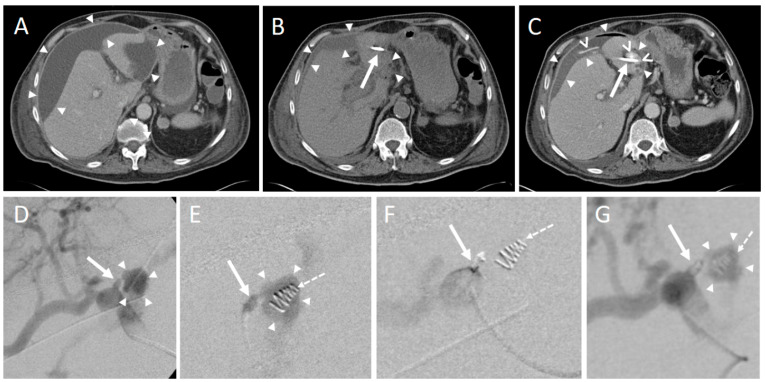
CT-guided drainage placement with a subsequent major complication according to SIR. (A) 71-year-old male underwent pancreaticoduodenectomy (PD) due to ductal pancreatic adenocarcinoma. Sixteen days later abdominal revision surgery with hematoma evacuation was performed due to arterial hemorrhage from the hepatic artery and small bowel segment resection due to adhesion ileus. Twenty-seven days after PD a perihepatic subcapsular fluid collection was diagnosed on a CT scan, mainly located at the ventrolateral and medial margins (arrowheads). (B) CT fluoroscopy-guided placement of a 10F Flexima^®^ single lumen drainage (arrow; Boston Scientific, Marlborough, MA, USA) within the fluid collection (arrowheads). After placement, a significant reduction of the fluid was observed. (C) Contrast-enhanced CT control scan after eleven days revealed an increase of the subcapsular fluid collections mainly next to the right liver lobe (arrowheads). Contrast medium extravasation occurred inferior to the left liver lobe (segment 3) from the left gastric artery in terms of active bleeding from this vessel. Contrast medium extravasation could also be found in the fluid collection (open arrowheads). Arrow: drainage. An angiographic examination (not shown) performed immediately thereafter did not provide the source of the bleeding. (D) Repeated angiography after another 6 days showed extravasation of the contrast agent (arrowheads) from the left hepatic artery (arrow). (E) Superselective embolization of the bleeding was performed by placement of an 18/4 (Cook Medical, Bloomington, Indiana, USA) Tornado^®^ microcoil in the vessel (arrow) supplying the hemorrhage. However, there was an initial misplacement of the coil (dashed arrow), which came to rest in the adjacent pseudoaneurysm (arrowheads). (F) Subsequently, placement of a second 18/4 Tornado^®^ coil was conducted in the vessel neck (arrow; initially misplaced coil: dashed arrow). (G) The final angiographic control did not show a remaining contrast agent extravasation. Arrow: Vessel neck with coil; arrowheads: hematoma; dashed arrow: initially misplaced coil. In conjunction with the clinical course, the case was considered to be an arrosion hemorrhage induced by the drainage placement in combination with insufficiency of the biliodigestive anastomosis.
